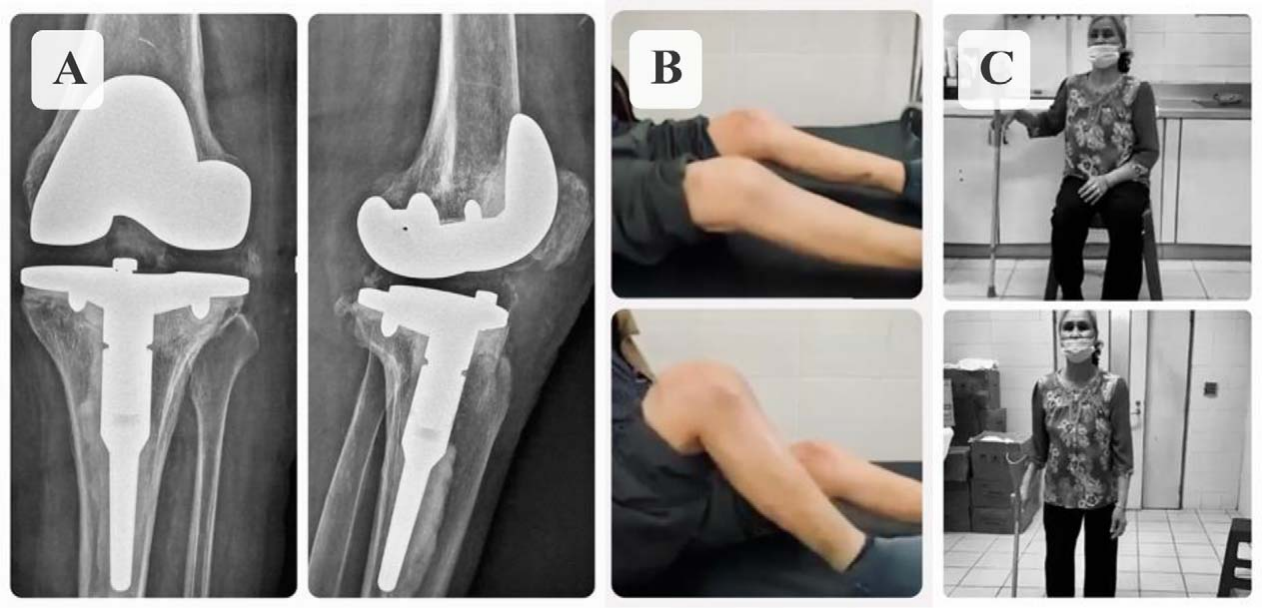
A. Postoperative X-ray of revision surgery by hinge prosthesis, B. Patient with cement spacer in place and C. Patient undergoes revision at final follow-up.
